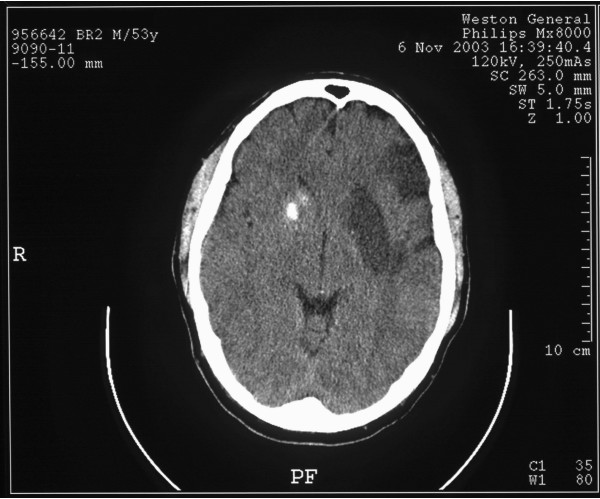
Computed tomography scan of the brain 72 hours after admission.
